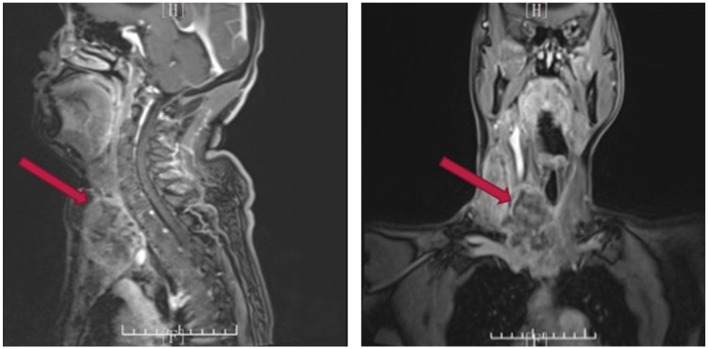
Cervical enhanced MRI indicating tumor recurrence.
